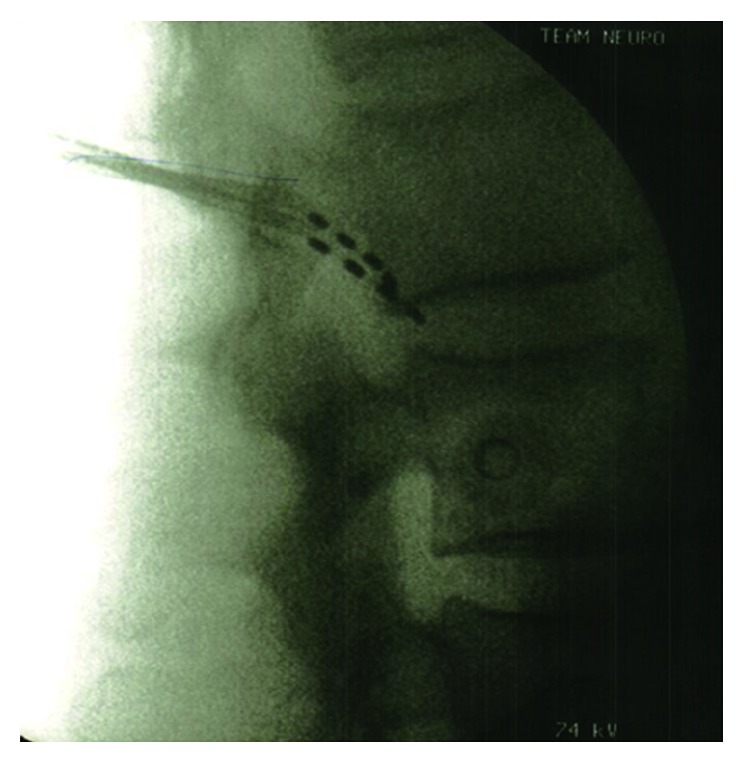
X-ray of lumbar spine, lateral view confirming the placement of electrodes.
